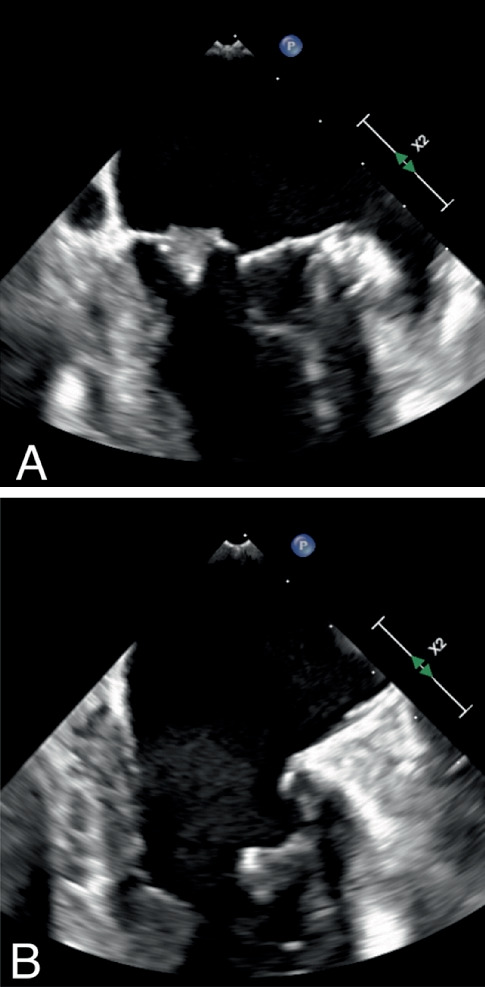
(A) Transesophageal echocardiogram demonstrating 1.2 × 1.0 cm vegetation on the posterior leaflet. (B) Transesophageal echocardiogram demonstrating 1.0 × 0.9 cm vegetation on the subvalvular apparatus of the anterior leaflet.
